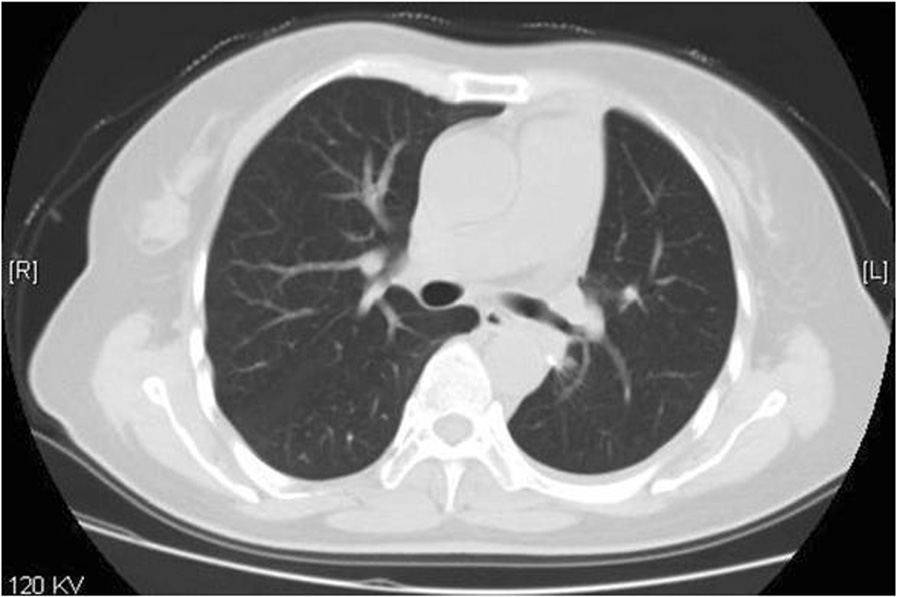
Chest CT scan at 1 month after operation
